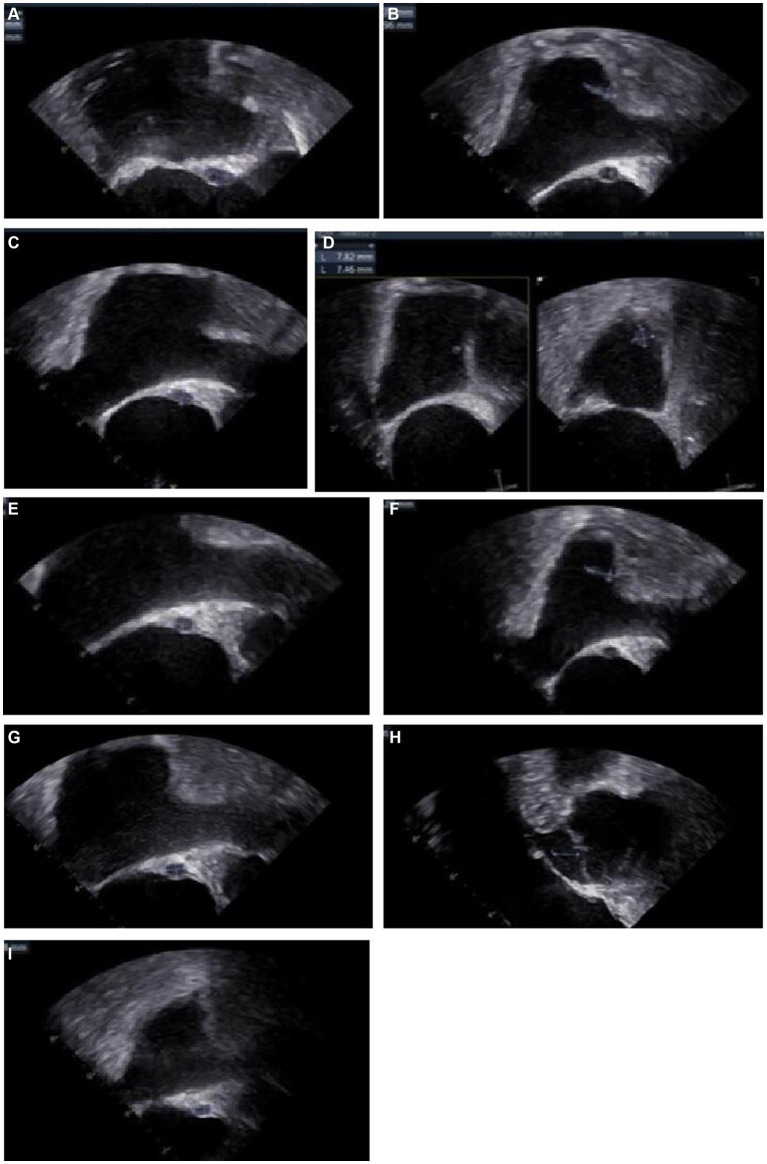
Images of transesophageal echocardiography (TEE) (A) Day 43, a fusiform heterogeneous hypoechoic mass (11.5*6.0 mm) in the atrial septum, and a fluid dark area could be seen. (B) Day 43, a stream-like echo (11.0*3.7 mm) at the entrance of the superior vena cava. (C) Day 55, the mass (10.6*5.7 mm) in the atrial septum. (D) Day 55, a moderate echogenic mass (13.0*5.9 mm, basal width 4.6 mm) near the opening of the superior vena cava, with a large amplitude of oscillation during the heart rhythm cycle. A moderate echogenic mass (9.5*7.0 mm, base width 6.9 mm) near the orifice of the inferior vena cava. (E) Day 101, the abscess (6.25*4.47 mm) in the atrial septum. (F) Day 101, the moderate echoic mass (12.3*3.7 mm, base width 3.4 mm) near the opening of the superior vena cava, the mass near the opening of the inferior vena cava disappeared. (G) Day 129, the heterogeneous hypoechoic mass (6.35*4.47 mm) in the atrial septum. (H) Day 129, the moderate echogenic mass near the opening of the superior vena cava was not seen. (I) Day 235, the heterogeneous hypoechoic mass (6.18*4.07 mm) in the atrial septum.
